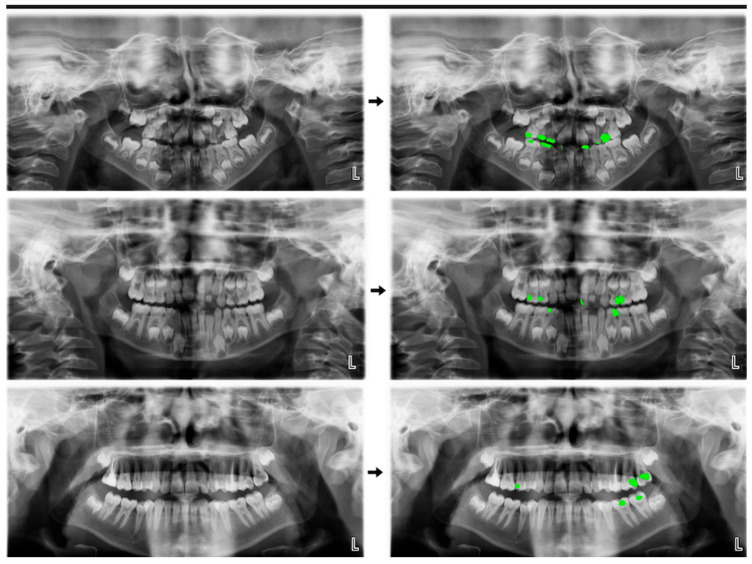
Caries segmentation using an AI model on panoramic radiographs of children with primary dentition, mixed dentition, and permanent dentition.
